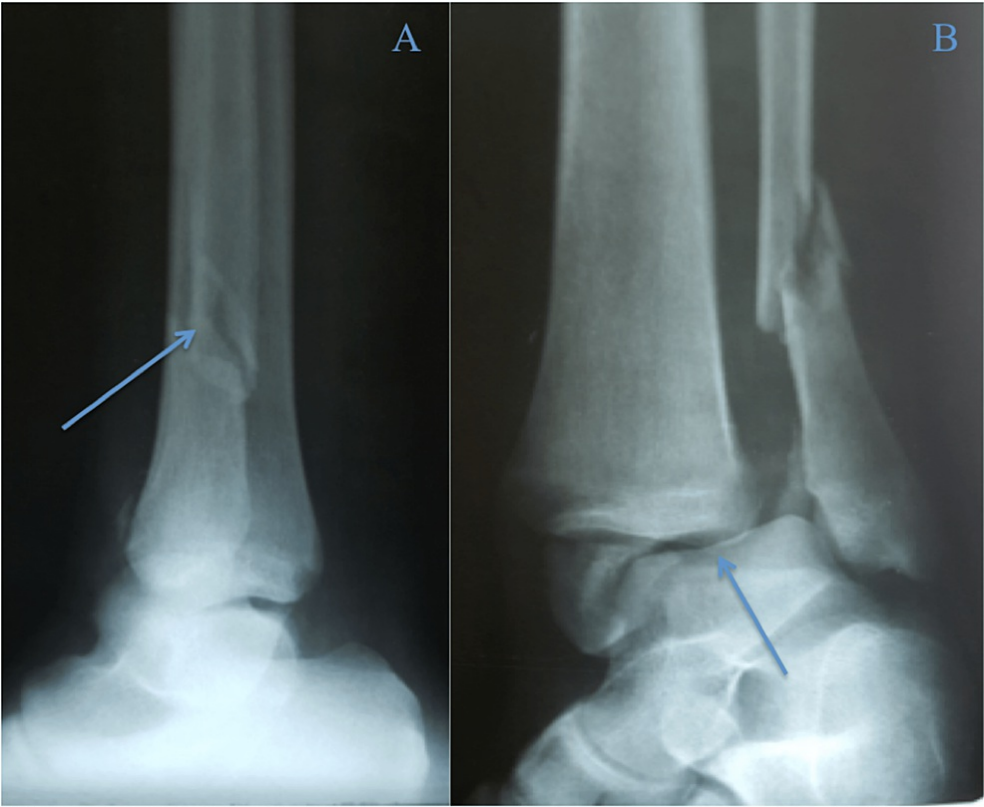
Plain radiographs (lateral and mortise views) Arrow descriptions: A - fracture site seen in lateral view, B - subluxation seen in mortise view
